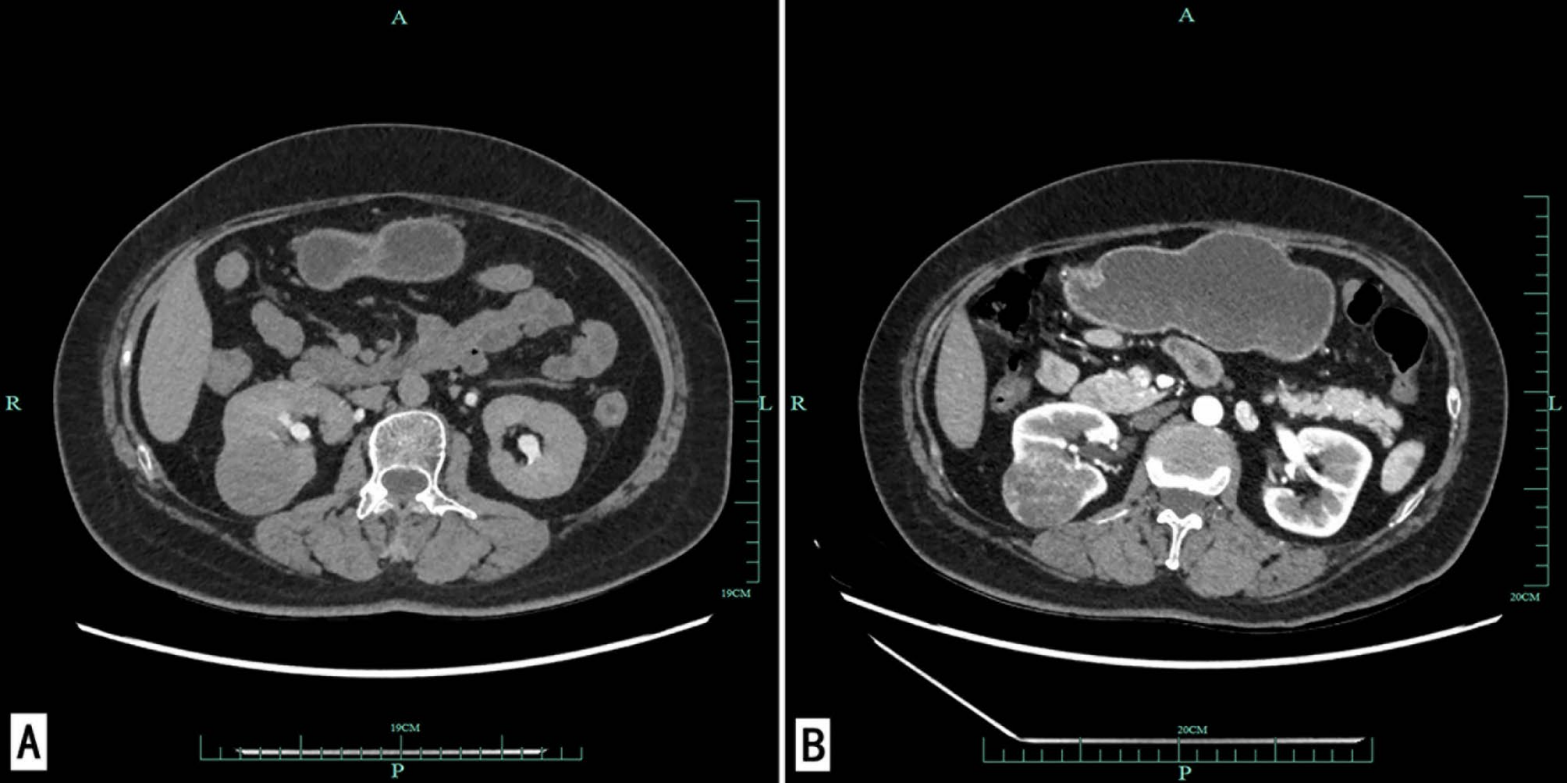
CT image of urinary system: A, the plain scan image showed a round soft tissue density shadow in the right kidney B, the enhanced image showed uneven and obvious enhancement in the cortical phase, and the degree of enhancement was slightly lower than that of the renal cortex
